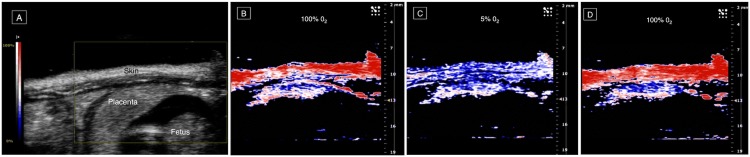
Photoacoustic (PA) imaging of placental oxygenation on day 14 of gestation. From left to right: (A) the placenta in a sagittal plane (obtained by a B-mode ultrasound scan) and parametric images created with the PA Oxyhemo mode making possible the evaluation of blood oxygen saturation during variations in the oxygen levels supplied to the mother (5–100%). PA imaging sequences during hyperoxygenation (B), hypoxia (C) and hyperoxygenation (D).
